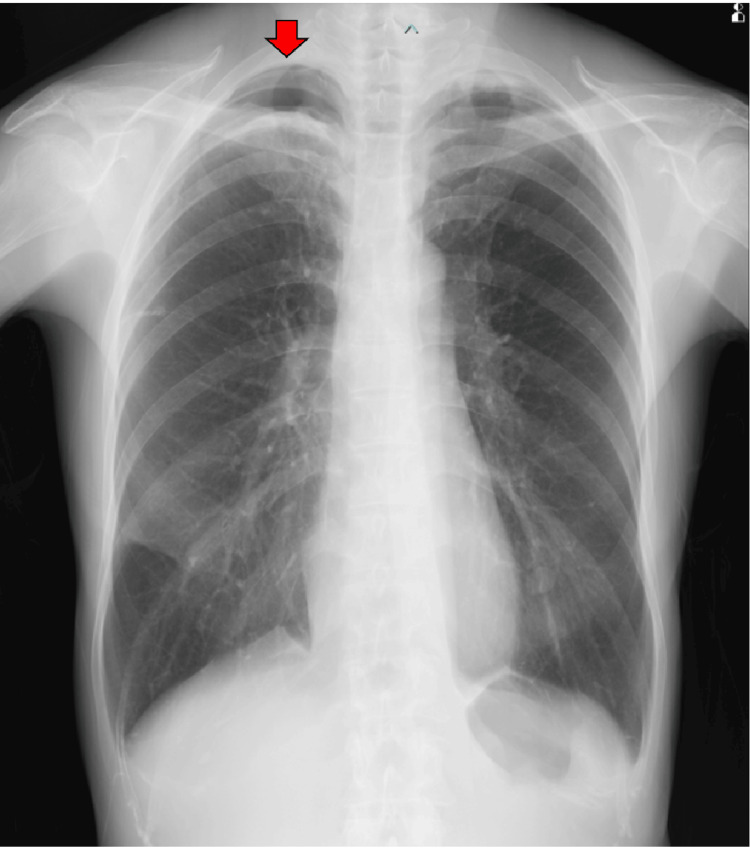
Chest radiograph on postoperative day five A space (red arrow) is present in the right lung apex, but lung expansion is preserved
